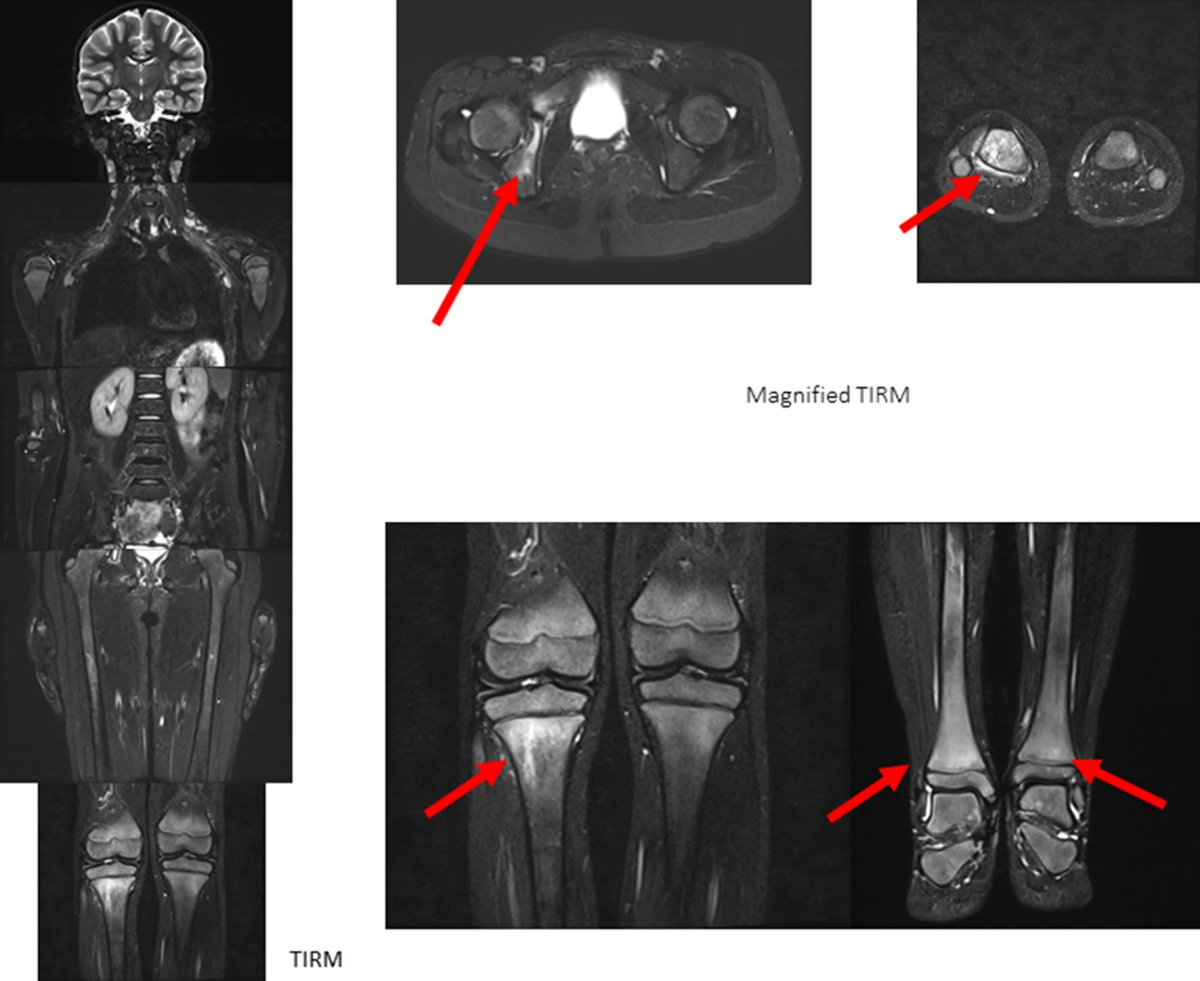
An 8-year-old girl without previous history of MSK pathologies and pain at multiple body regions (back, lower extremities). MRI shows bone marrow edema, affecting the long bones (with focus on epiphyseal and metaphyseal areas) and pelvis (acetabulum)
